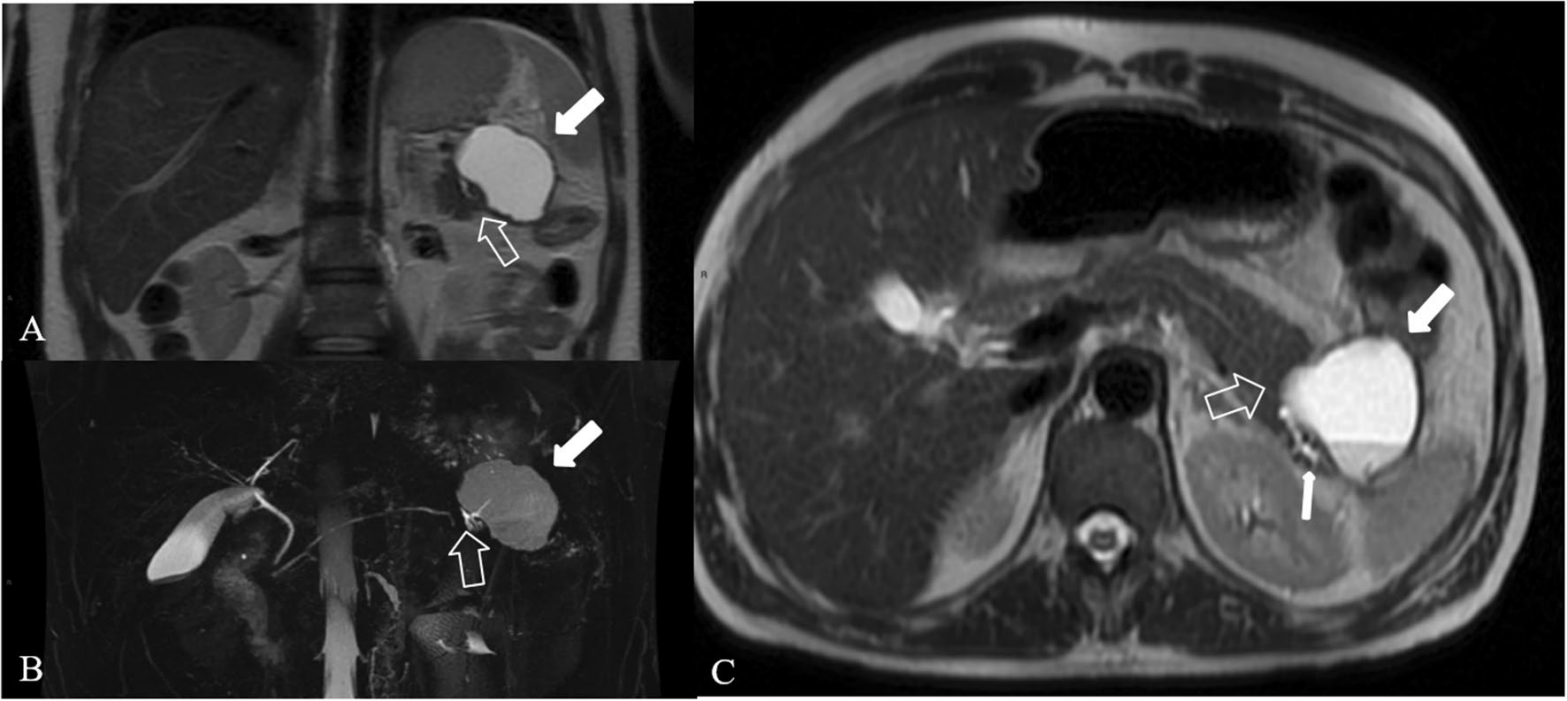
Disconnected pancreatic duct syndrome in a 41 years-old man. Coronal (a), axial (c) T2 weighted MR images and MRCP (b) 3 weeks after the onset of the symptoms show focal interruption of the Wirsung duct (empty arrow)—upstream pancreatic duct dilatation (thin arrow in c)—and a heterogeneous encapsulated fluid collection in the pancreatic tail (white arrow)
